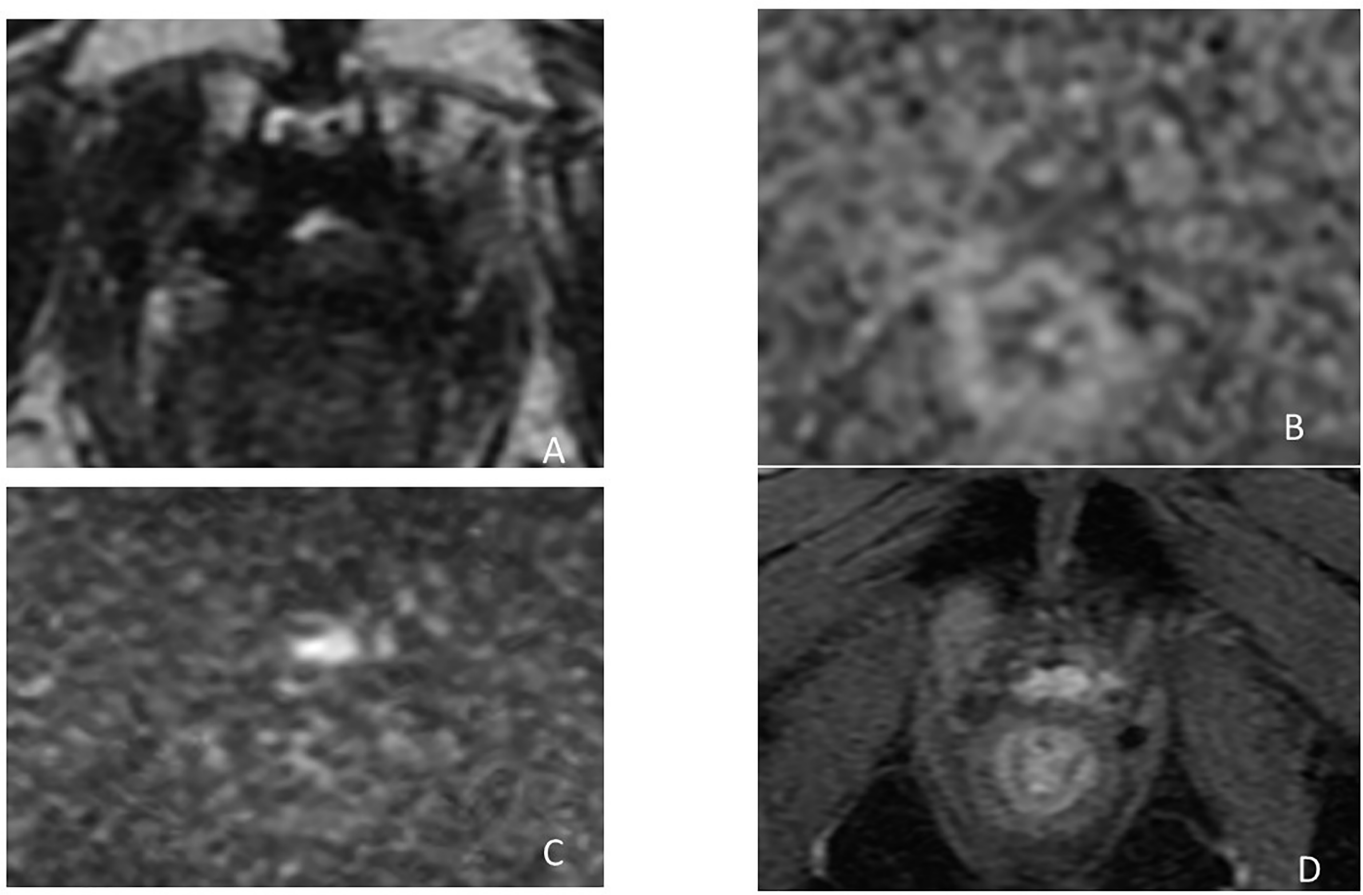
Postsurgical lesion recurrence (arrows) in a 63-year-old male with serum PSA = 0.6 ng/ml after radical prostatectomy. Axial T2W MRI (A), ADC map (B), b2000 DW MRI (C), and DCE MRI (D) show a soft tissue lesion at the posterior part of VUA, relatively hyperintense on T2W best seen on high *b*-value and DCE imaging with a focal nodular enhancement that contrasts sharply with the general background low-level venous enhancement.
